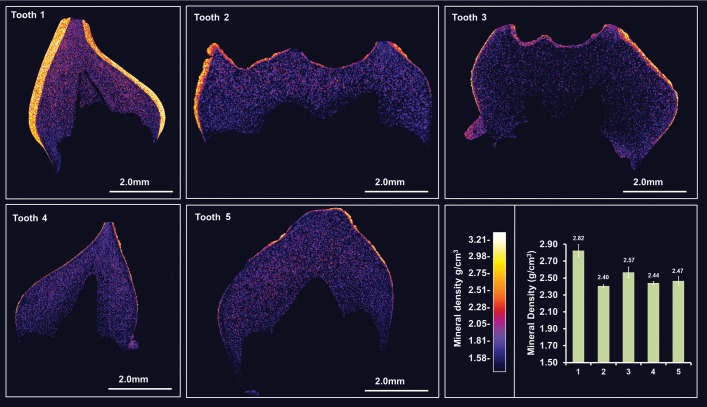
High resolution X-ray CT analysis of exfoliated teeth 1–5. Typical CT sections through the teeth are presented using false colour calibrated with respect to mineral density to generate mineral density maps. Mean enamel mineral density for each tooth is also shown graphically. Tooth 1 exhibits an enamel layer apparently normal in structure and density. Teeth 2 and 3 exhibit an obvious enamel covering though it is thinner in many areas, chipped, absent in places and is significantly reduced in mineral density compared with tooth 1 (*P* < 0.0001). Teeth 4 and 5 exhibit an even thinner covering of enamel with enamel absent in some areas. Again enamel mineral density is significantly lower than in tooth 1 (*P* < 0.0001). The only non-significant differences in enamel mineral density occur between teeth 4 and 5. Videos of 3D rendered CT data showing surface detail and internal structure of all teeth are available as Supplementary Material.
